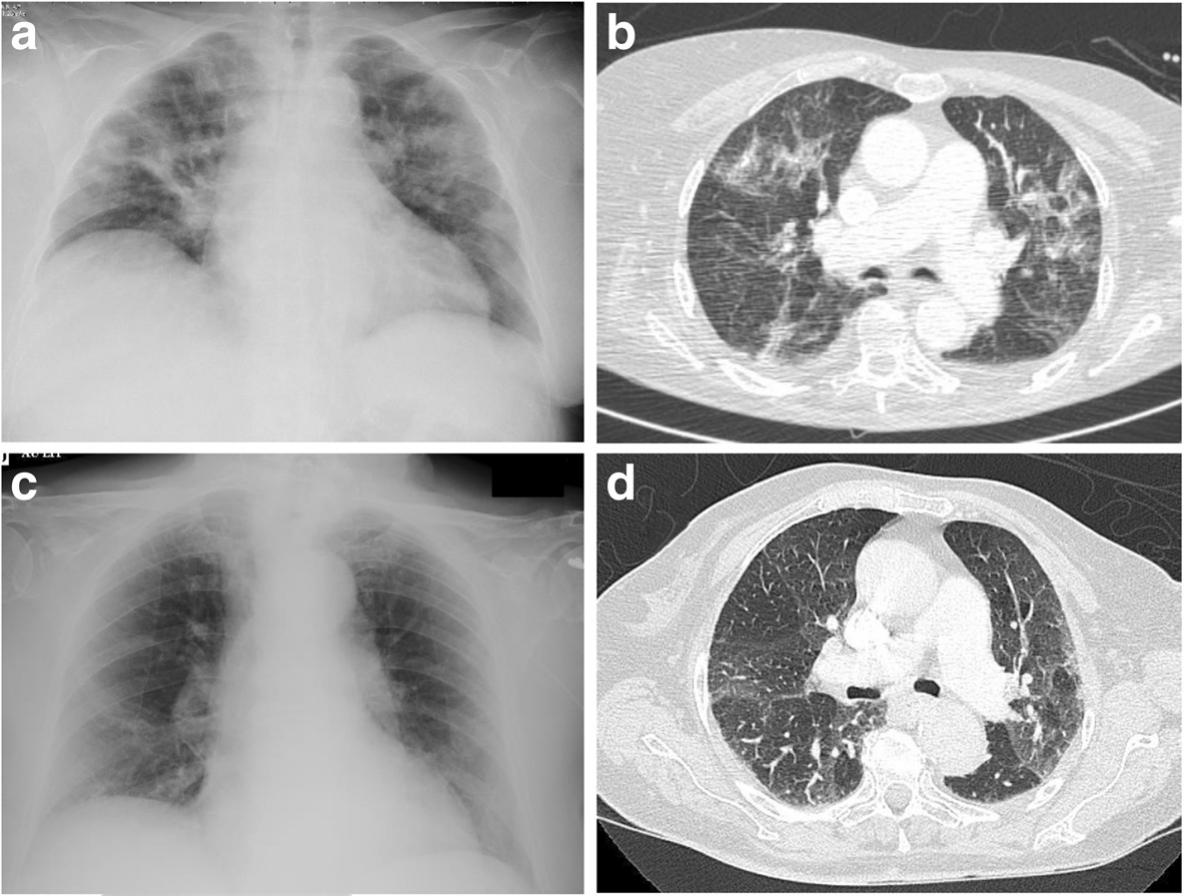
Chest X-ray (a, c) and computed tomography (b, d) for the two patients with eosinophilic pneumonia
